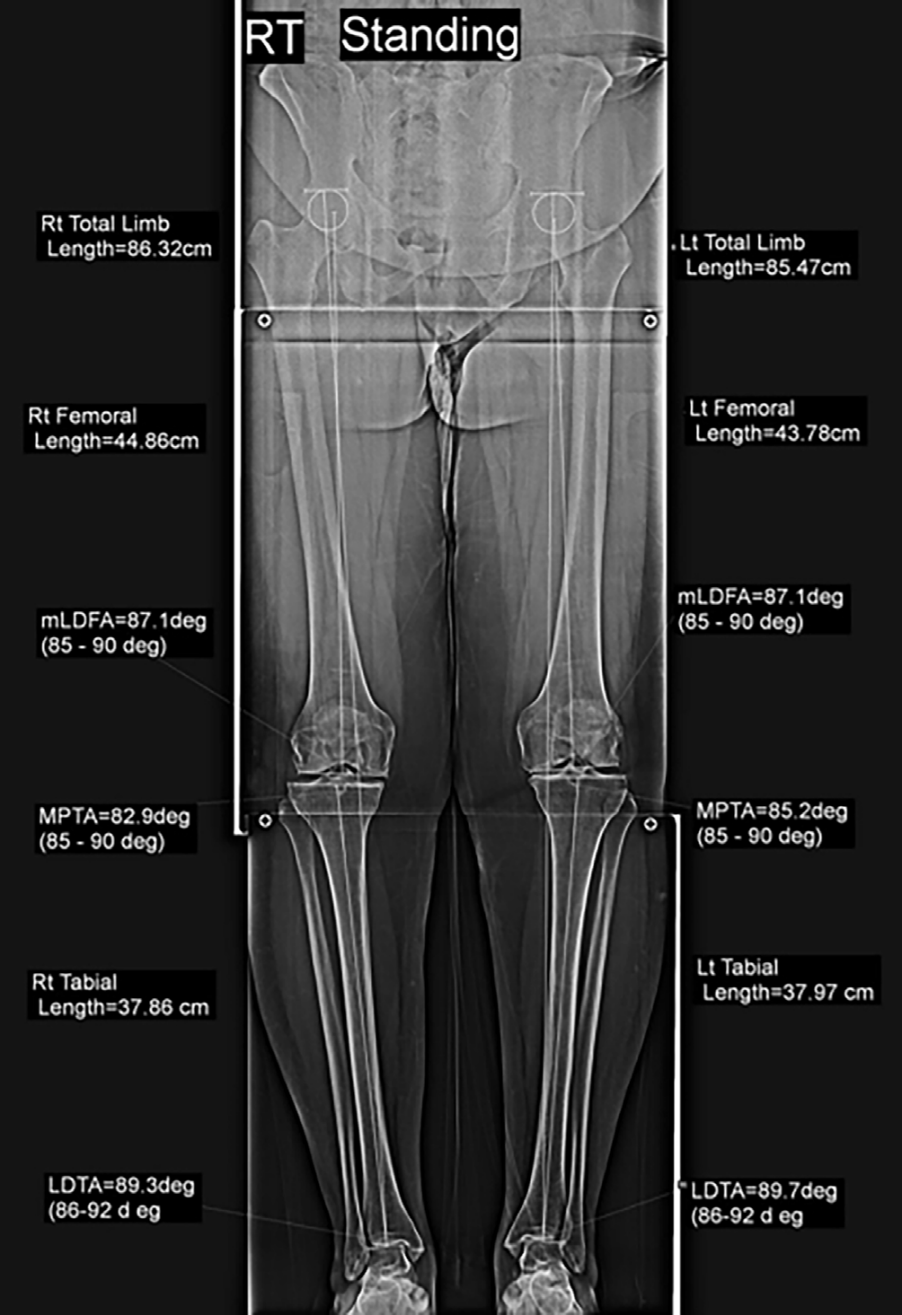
Preoperative planning using standing whole lower limb radiograph.
